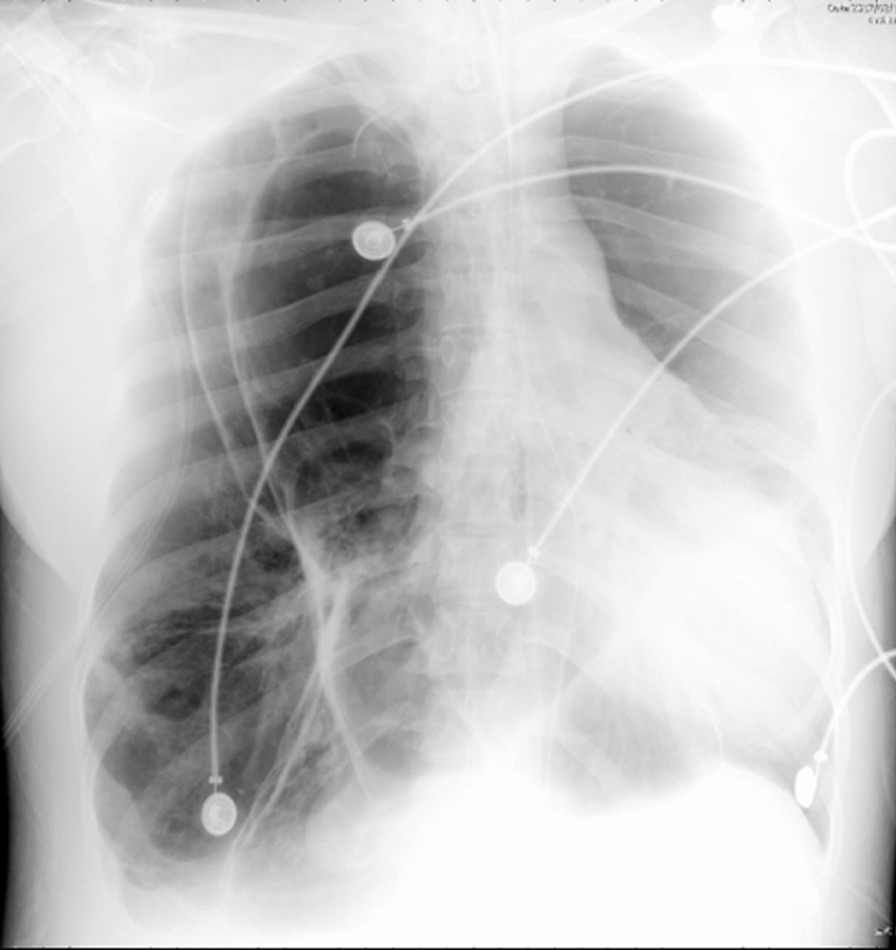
Chest X-ray revealing increased radiolucency of almost the right lung, and the mediastinum shifted to the left
